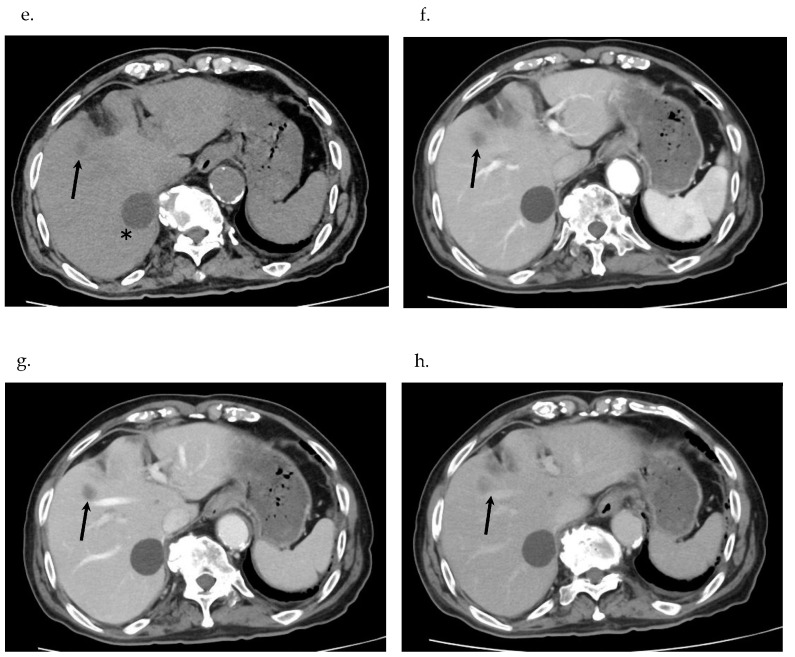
Ultrasonography (US). (a) B-mode Plain US, a 15 mm taro-shaped hyperechoic nodule with halo in S5 (arrow). (b) Contrast-enhanced US (CE-US), hypervascularity in the early vascular phase in S5 (arrow). (c) CE-US, early washout in the portal phase in S5 (arrow). (d) CE-US, defect in the postvascular phase in S5 (arrow). Computed Tomography (CT). (e) Plain CT, a 15 mm hypodense nodule in S5 (arrow) and a 45 mm hepatic cyst in S7 (asterisk). (f) Contrast-enhanced (CE-CT), hypovascularity in the early phase in S5 (arrow). (g) CE-CT, no enhancement in the portal phase in S5 (arrow). (h) CE-CT, the equilibrium phase in S5 (arrow).
